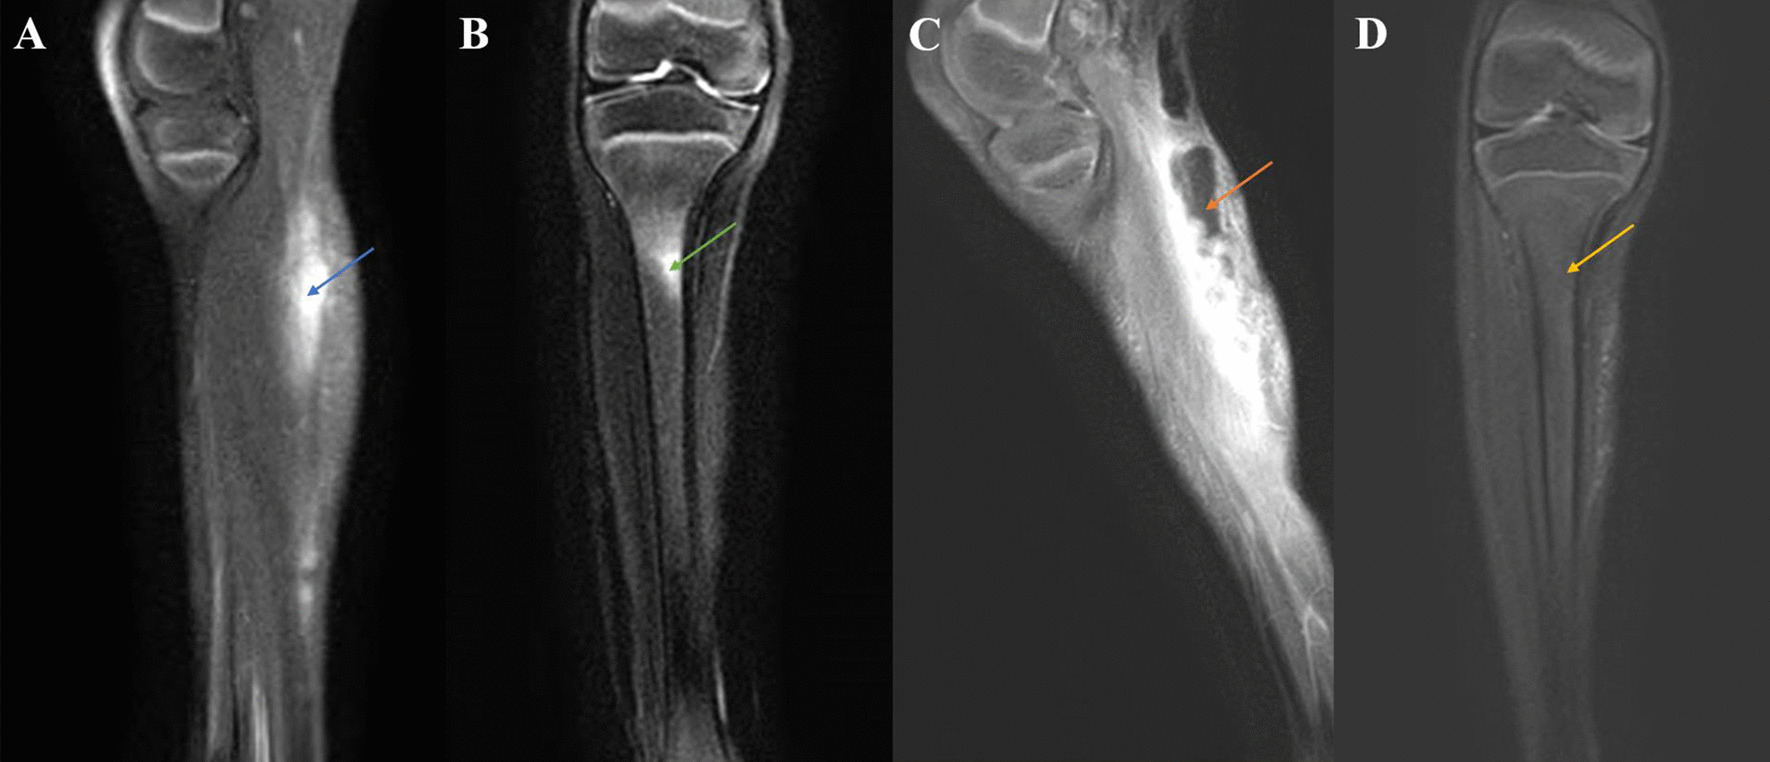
MRI findings of the right lower extremity. A Sagittal view of the right lower extremity MRI scan showing abnormal signal shadows (blue arrow) in the cutaneous and subcutaneous areas. B Coronal view of the right lower extremity MRI scan showing tibia bone marrow edema (green arrow). C Sagittal view of the right lower extremity MRI scan showing that the infection of the soft tissue had progressed, involving the right gastrocnemius muscle with pus cavity formation (orange arrow). D Coronal view of the right lower extremity MRI scan showing that the tibia bone marrow edema had been resorbed (yellow arrow)
